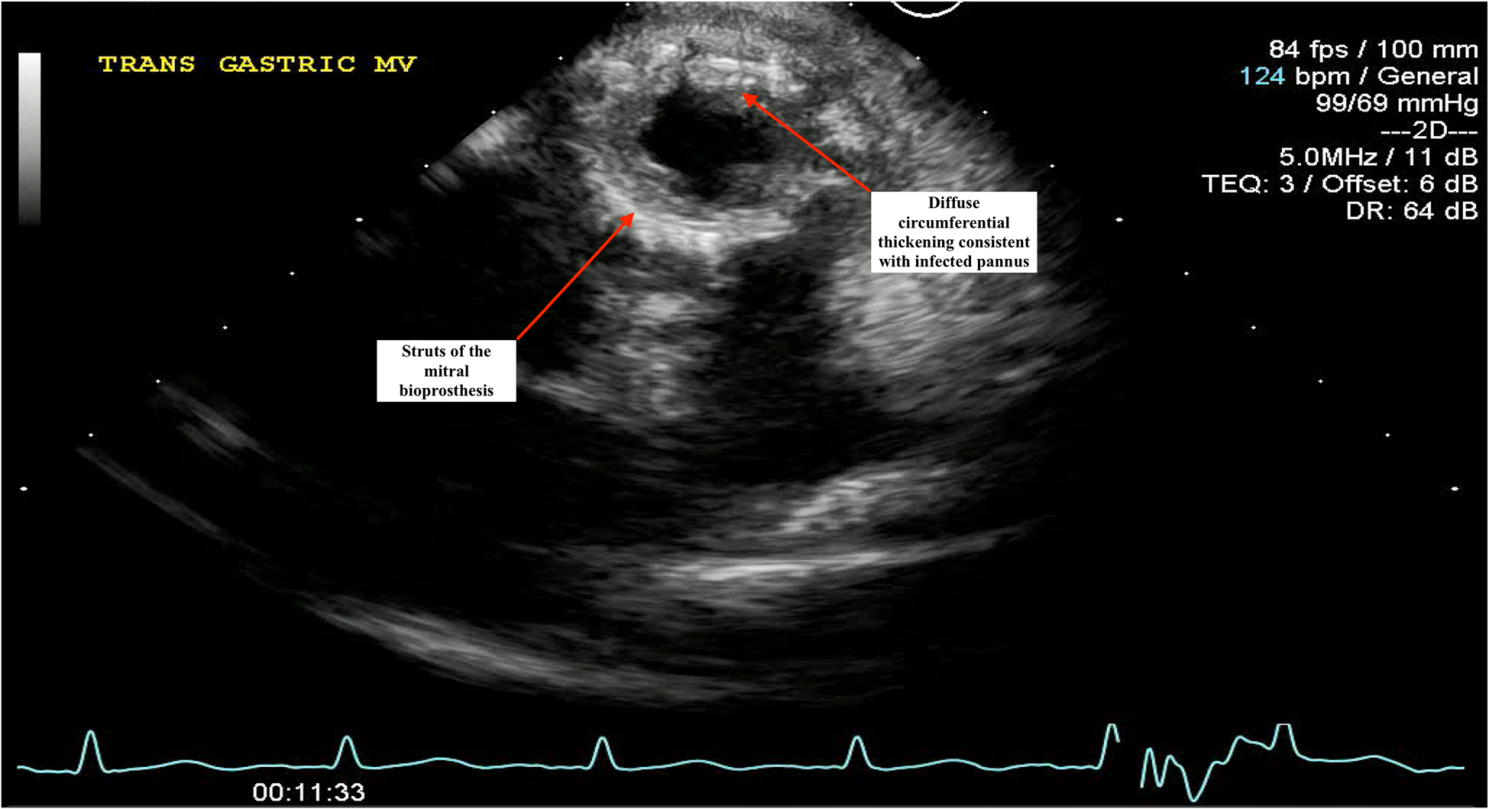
Transesophageal transgastric short axis view of the infected mitral bioprosthesis with diffuse thickening of the bioprosthetic leaflets and extensive infected pannus resulting in narrowing of the orifice, explaining the mitral stenosis
